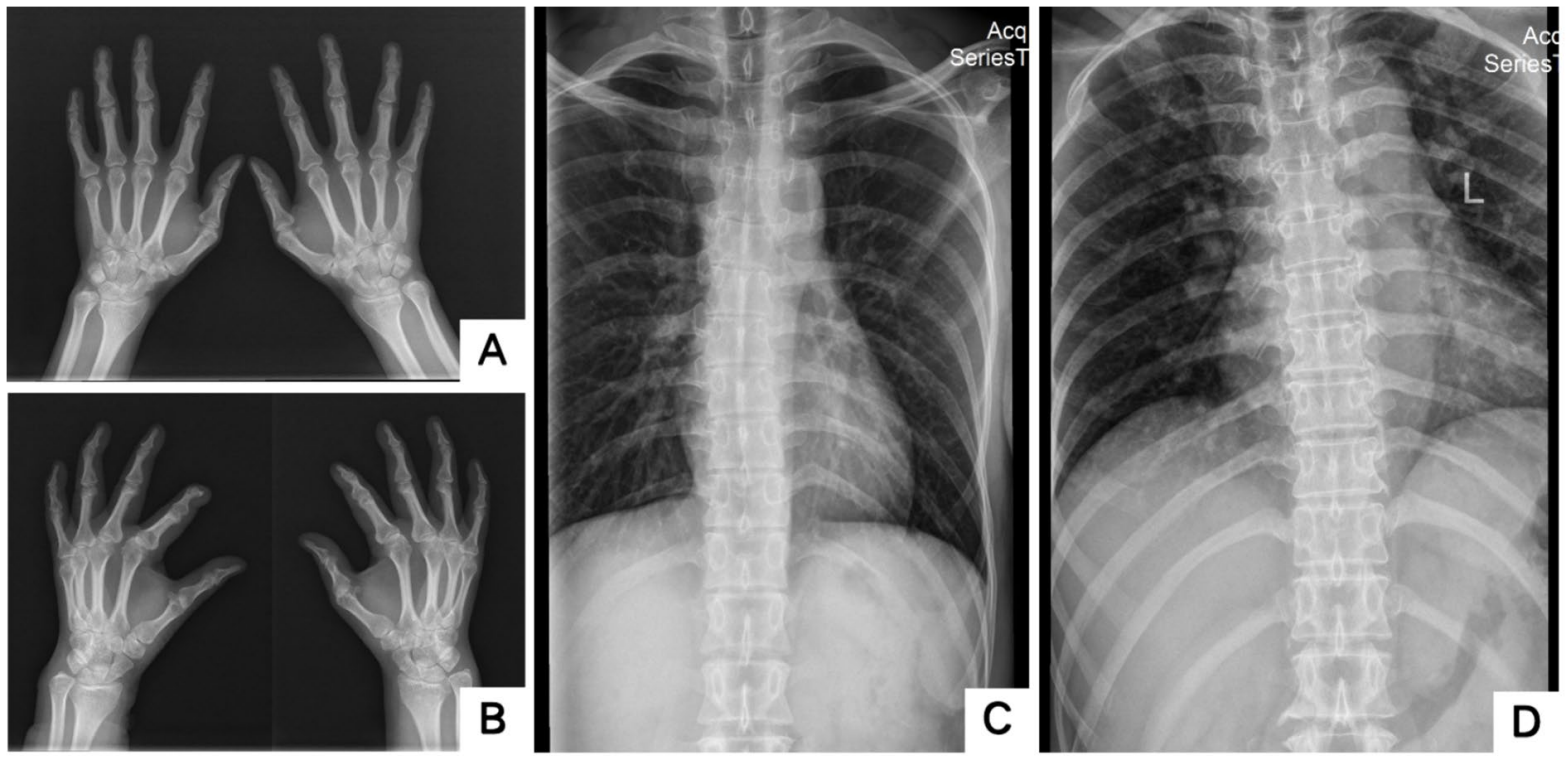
X-rays of two probands’ hands and scoliosis. A showed bilateral fifth-digit clinodactyly; B showed flexion deformity of the phalangeal joint of the left index finger; C,D showed Mild scoliosis (A,C: Proband 1 III-5; B,D: Proband 2 II-3).
